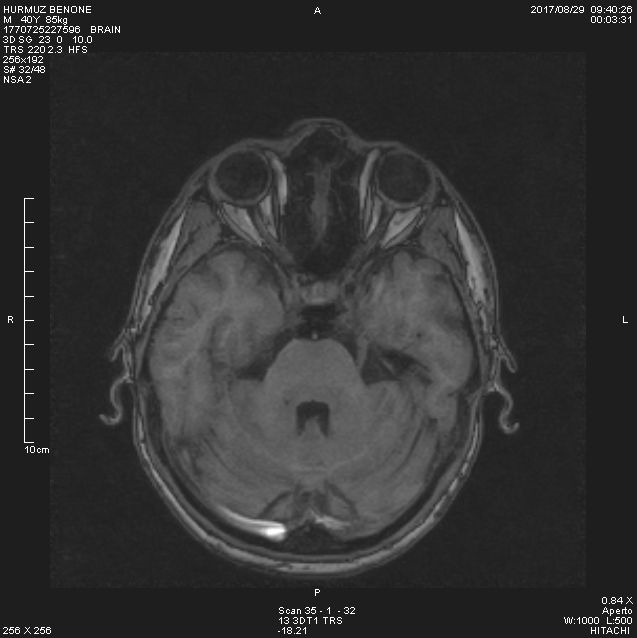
MRI HB - The MRI in our patient showed that the mean values of optic nerve volumes were significantly lower
